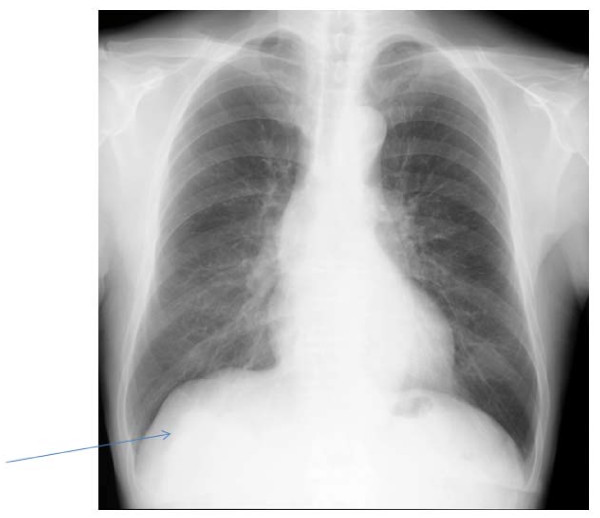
A chest x-ray revealed a mass measuring 65 mm in diameter in the right lower lung field.
